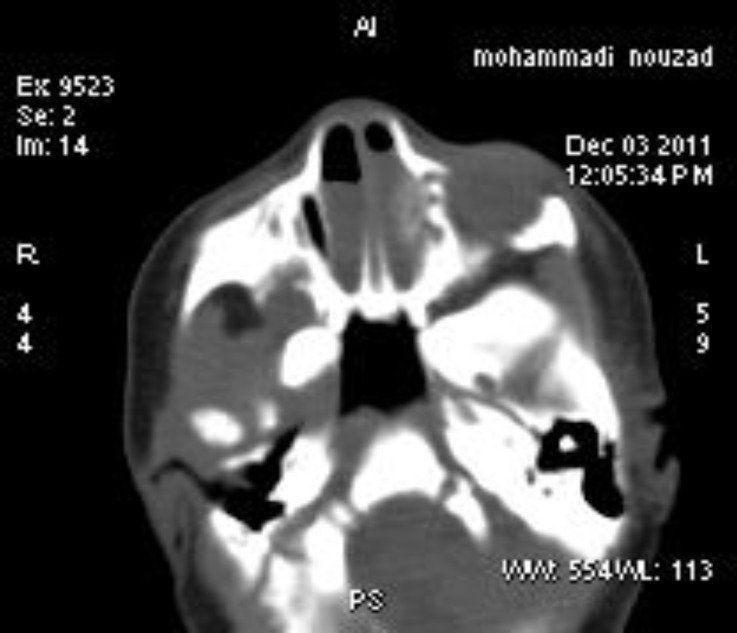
CT- Scan of bilateral choanal atresia
